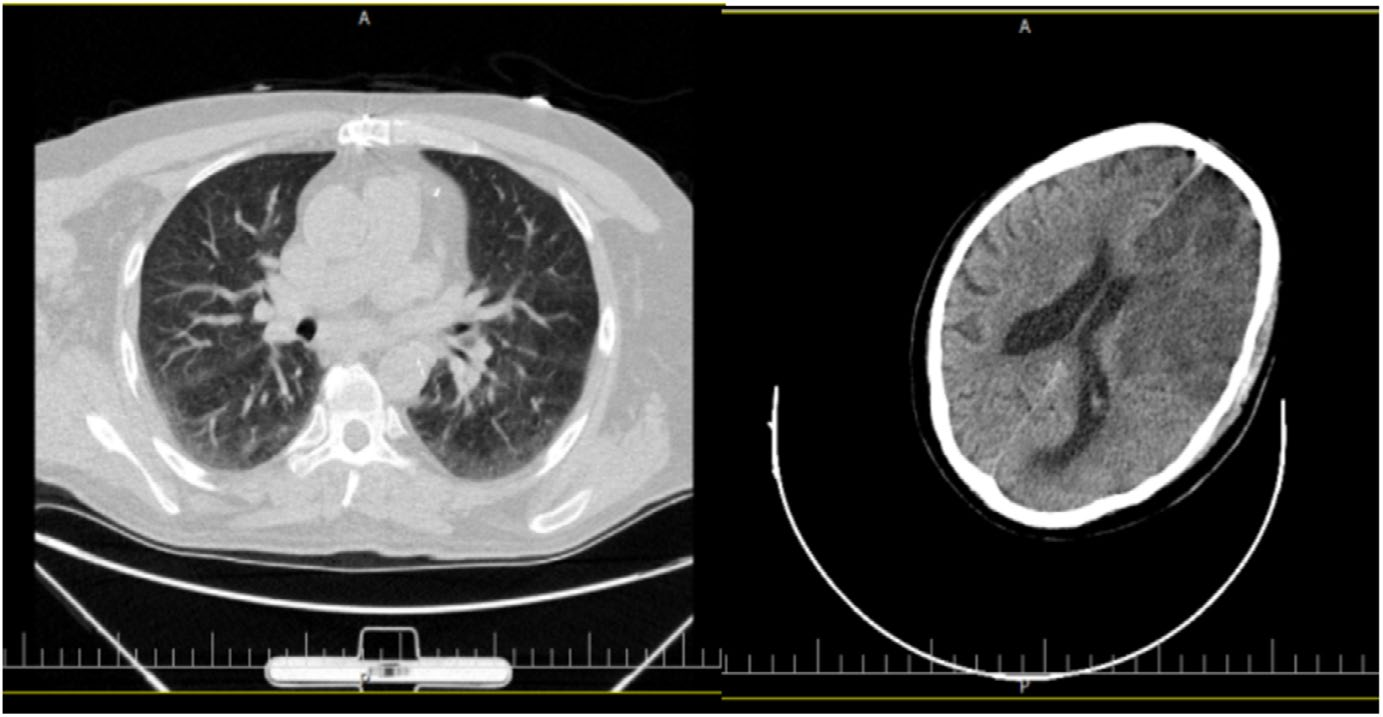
Chest and brain computed tomography.
